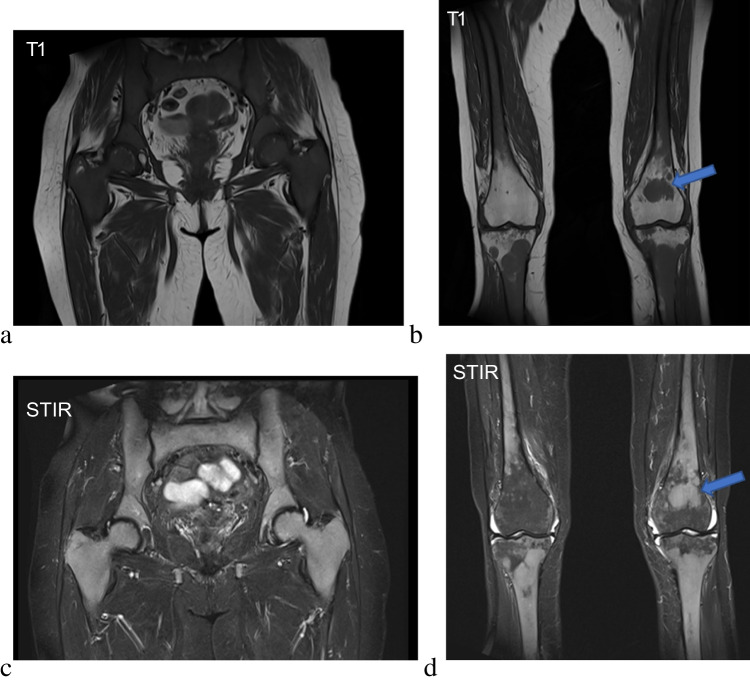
Seventy-three-year-old female with myelodysplastic syndrome. T1-weighted images of the pelvis and femora (a, b) demonstrate diffuse infiltrative appearances of the marrow with corresponding hyperintensity of the marrow on STIR sequences (c, d). Unlike SABM, the adjacent subcutaneous tissues are not involved in malignancy and are appropriately fat suppressed on fluid-sensitive sequences. Therefore, malignancy represents an important differential diagnosis of diffuse bone marrow signal change, but without the characteristic soft tissue signal abnormalities seen in SABM. The axial distribution is also suggestive of malignancy rather than SABM. The focal lesion in the left distal femoral medullary cavity (arrowed) is focal in nature, which is more in keeping with malignant infiltration, compared to SABM where changes are more diffuse
